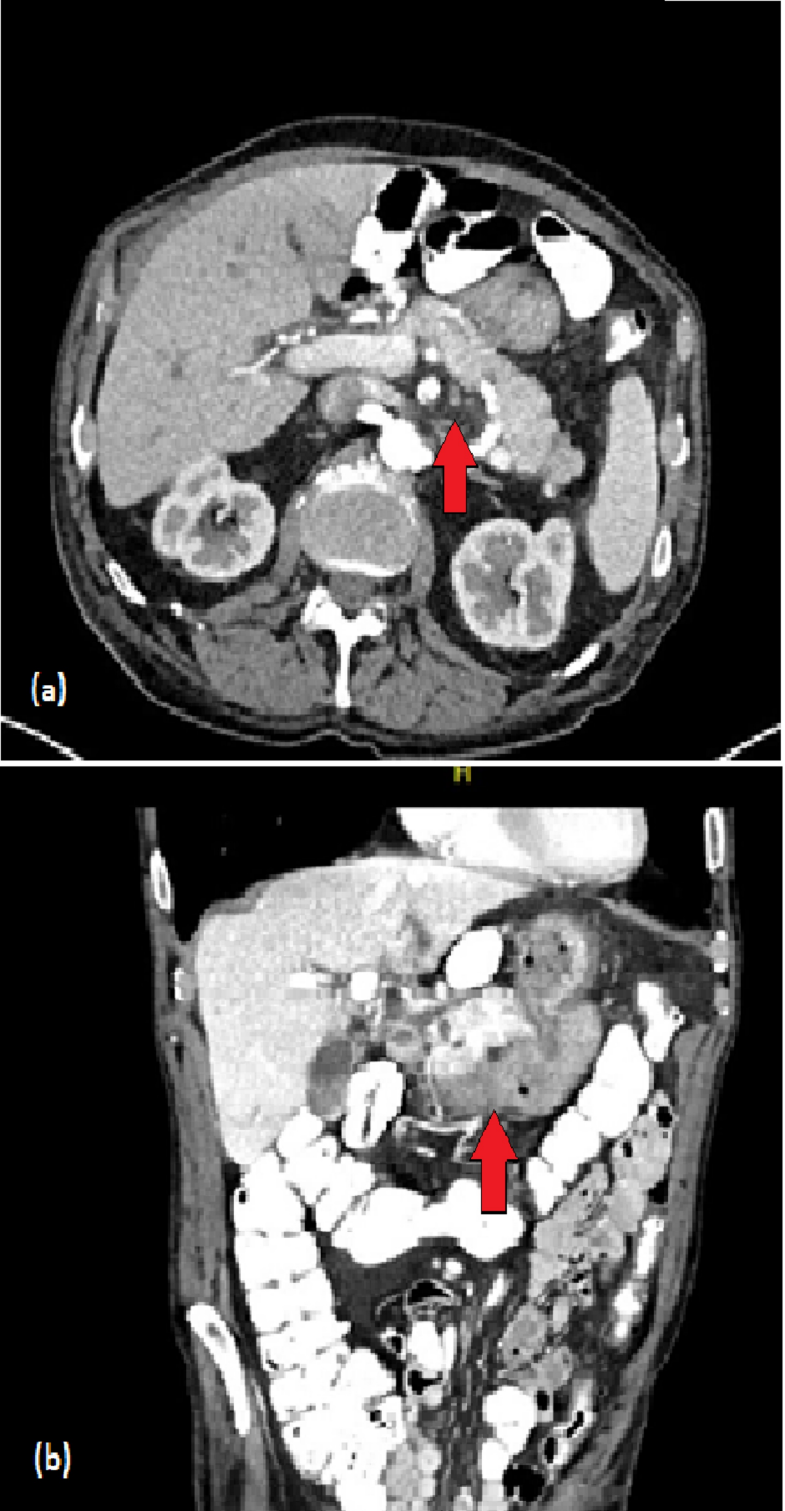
Follow-up computed tomography abdomen. Findings consistent with chronic pancreatitis within the head/uncinate of the pancreas (red arrows). The cystic lesions in the head and tail of the pancreas have continued to decrease in size. The pseudocyst abutting the wall of the stomach has resolved. (a) Axial view. (b) Coronal view.
